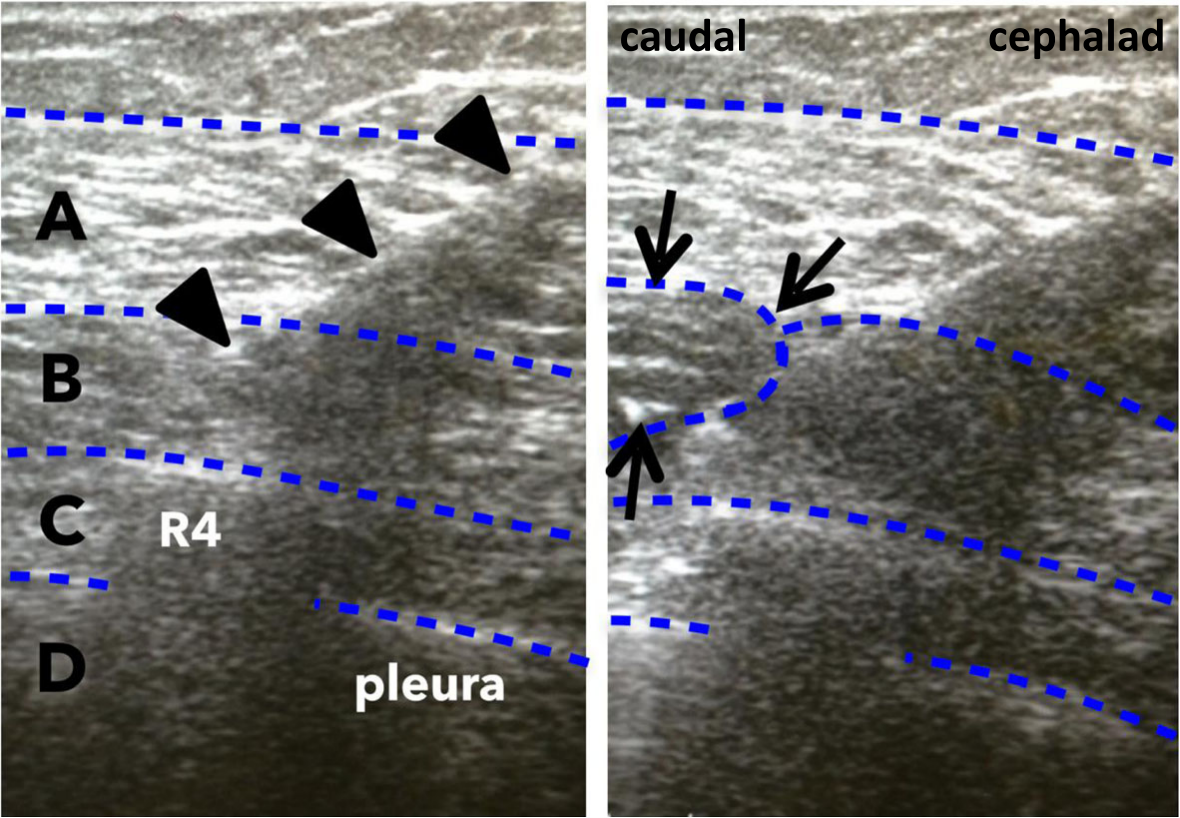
Ultrasound-guided serratus plane block
